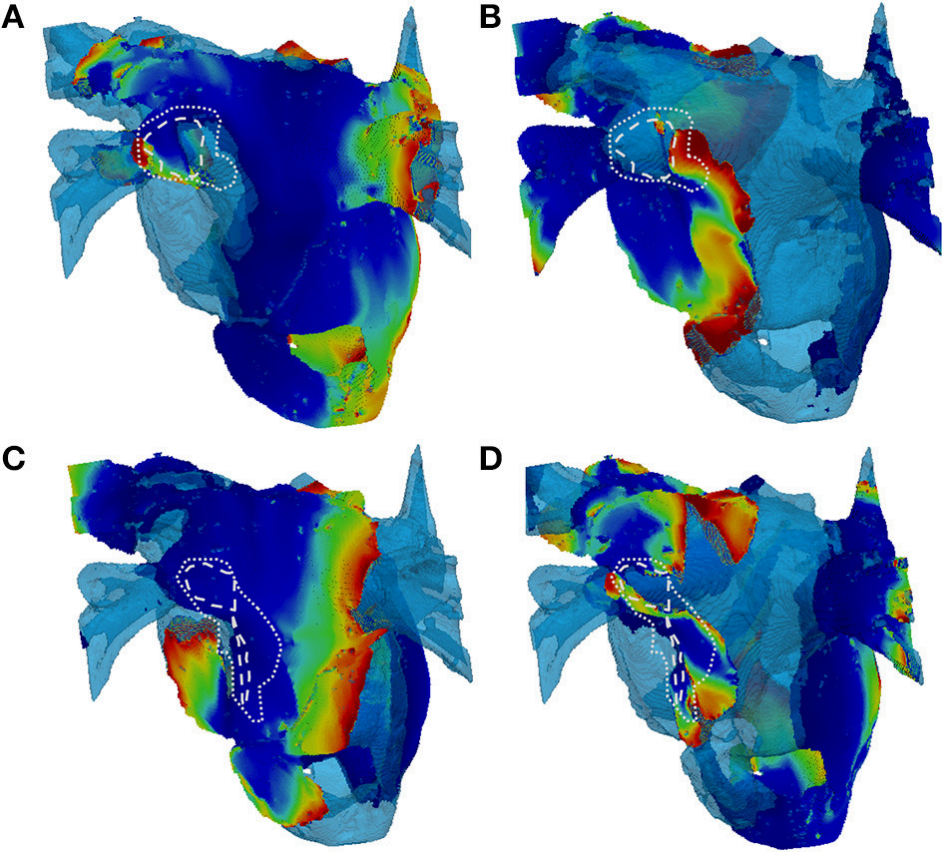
Snapshots of re-entry in 3D atria with fibrosis distributions based on high and low thresholds of LGE MRI intensity datasets. (A,C) Show 2 patient cases with a high threshold (lower amount of fibrosis) and (B,D) Show the respective cases with a low threshold (higher amount of fibrosis). The rows delineate different patients (A–D). In the high threshold cases (A,C), only a short lived rotor (indicated) was present in the LA. In the high threshold cases (B,D), multiple re-entrant waves are present in the LA fibrotic regions. White dashed and dotted lines indicate level 5 (dense) and levels 3–4 (patchy) levels of fibrosis, respectively. 3D voltage maps are superimposed onto the atrial geometry (see color code in Figure 6).
